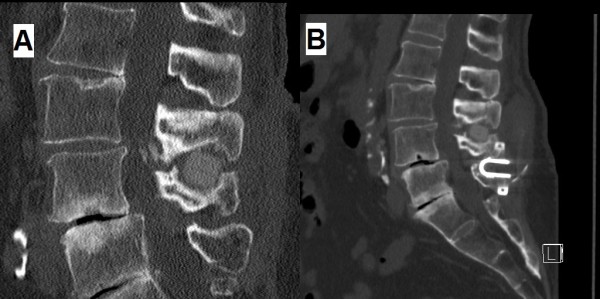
Case example of wrong level spine surgery. Sagittal reformatted CT scan of the lumbar spine; this patient came to our attention after having performed an ‘interspinous stabilization L4-5’ elsewhere, as reported in medical discharging chart; soon after surgery her bilateral *claudicatio radicolaris *started to worsen. A. the preoperative study clearly shows the DIAM^®^ interspinous device applied at the L3-4 interspinous space (wrong). B. in the postoperative image the COFLEX^®^ device correctly inserted at L4-5 is visualized; the DIAM^®^ at L3-4 was intentionally left in place to avoid late compromise of segmental stability.
